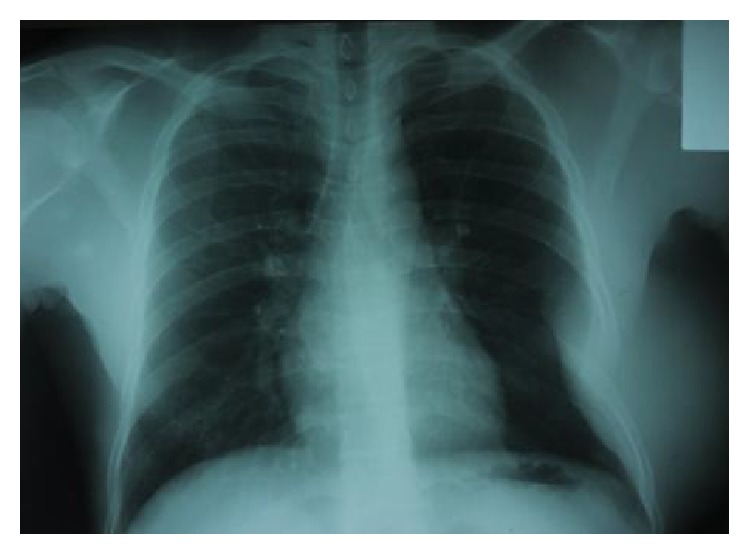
Chest X-ray showing unilateral peripheral opacity.
